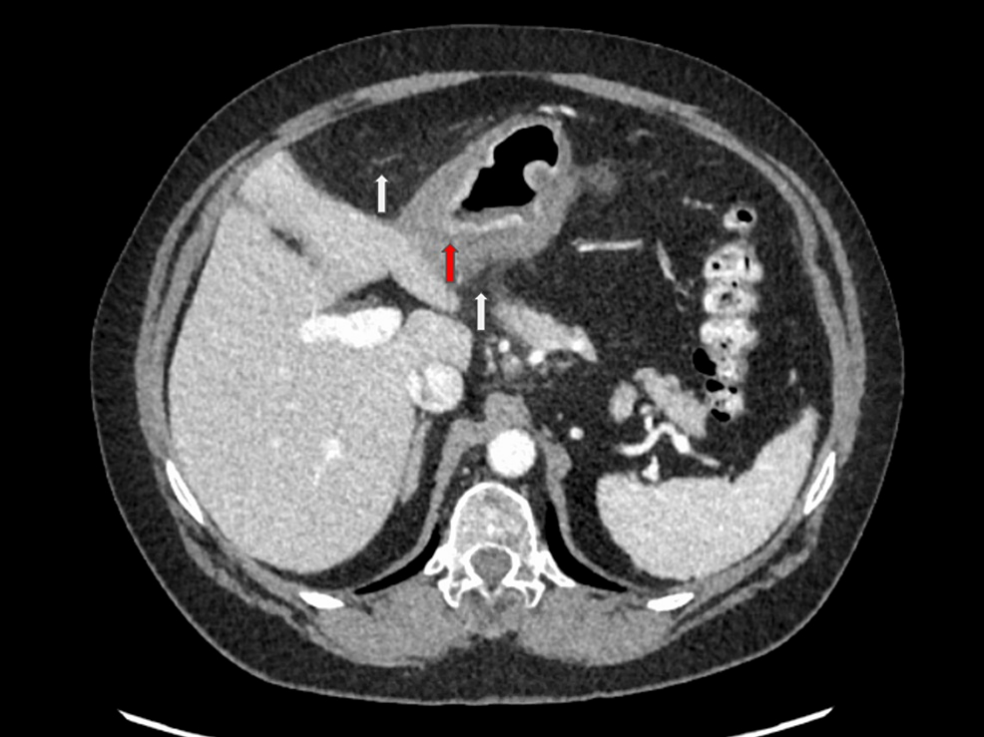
Axial CT of the abdomen and pelvis with contrast: perigastric fat stranding (white arrows) and concentric gastric wall thickening (red arrow) are both present as a result of inflammation. CT: computed tomography
